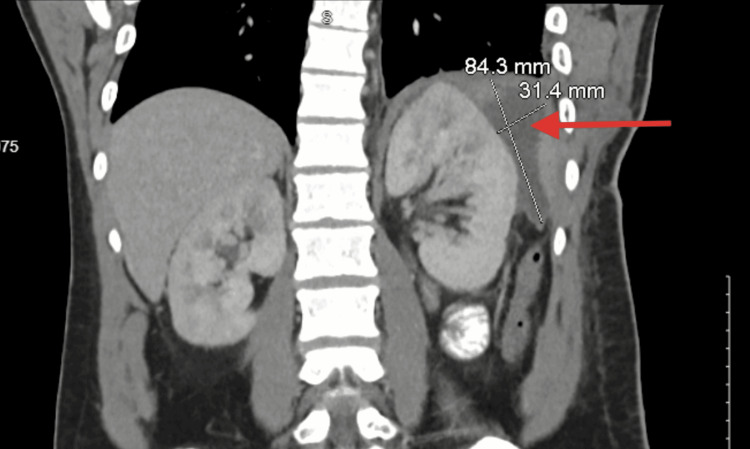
CT abdomen/pelvis showing a multiloculated subphrenic and perisplenic collection (red arrow)
